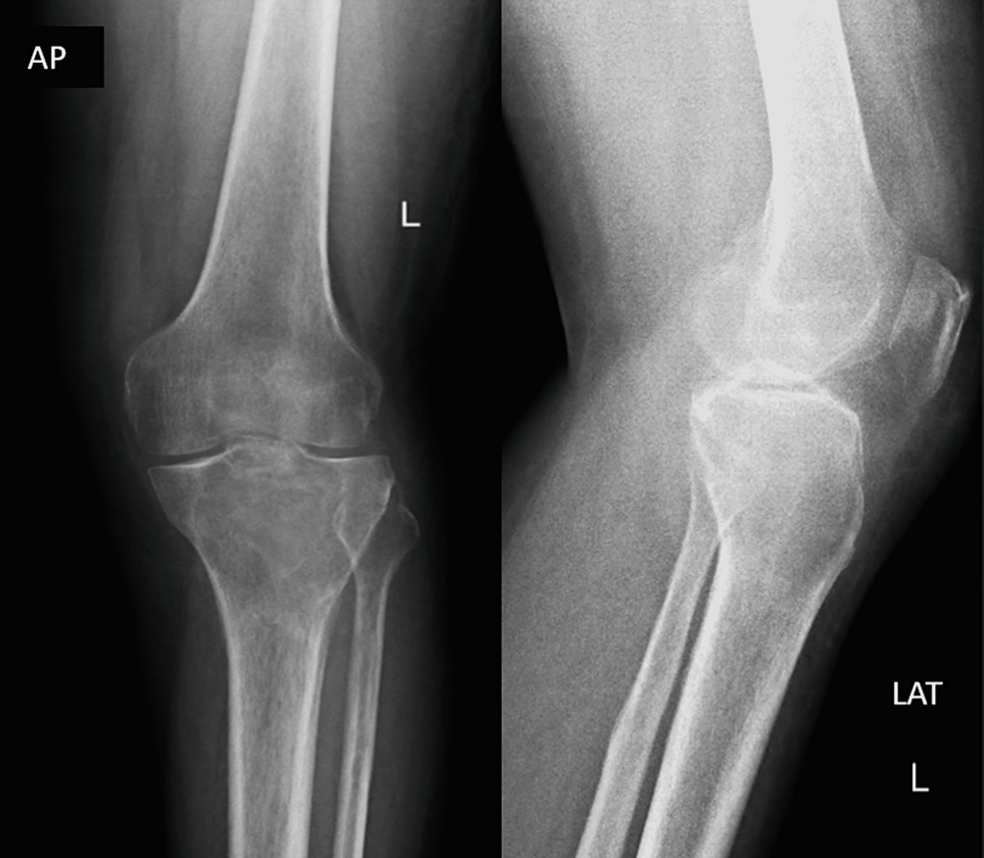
Radiograph suggests a lytic lesion in the proximal aspect of the tibia involving the epiphyseal-metaphyseal junction
